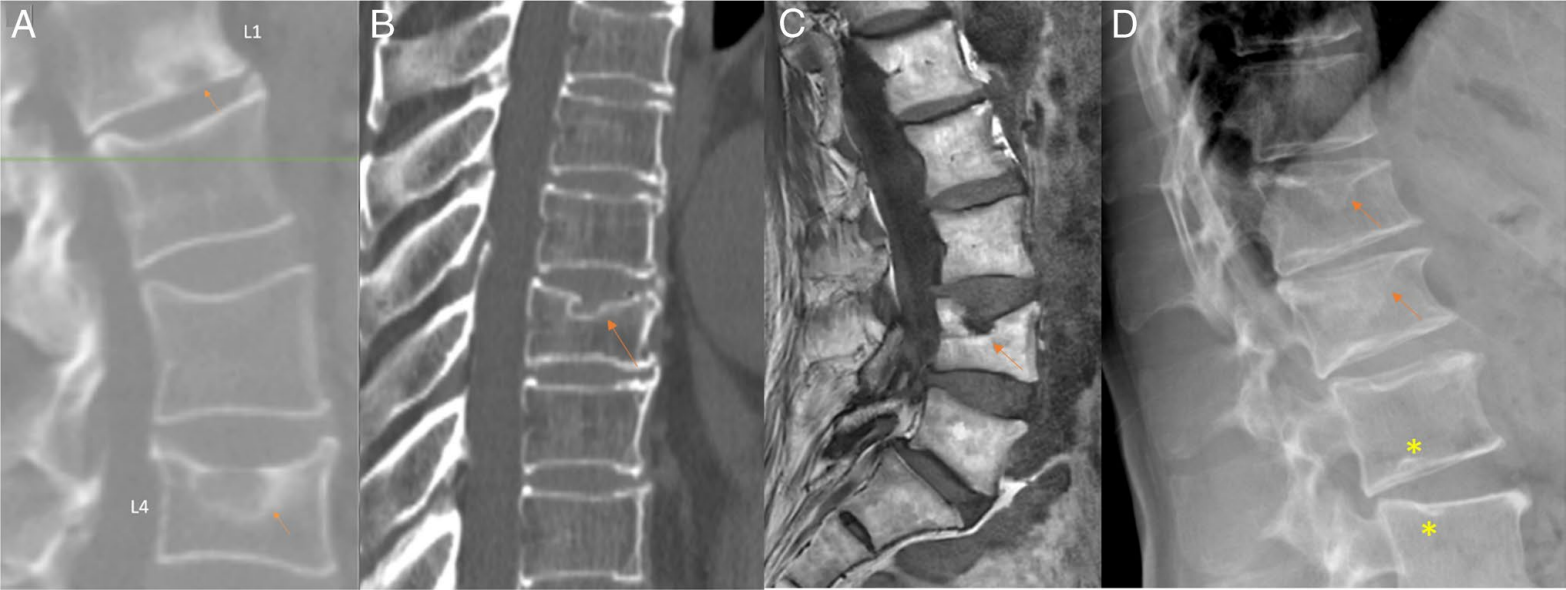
Image examples of Schmorl’s nodes of acquired cause (SNa). A Lateral radiograph. There are reactive bone changes in the inferior endplate of L1 (arrow: SNa), and a SNa in the superior endplate of L4 with an associated depression. B Reconstructed CT image. The arrow indicates the superior endplate SNa. C On T1-weighted sagittal MR image, the L4 OLVF with apparent superior endplate depression and the SNa are demonstrated. D Lateral radiograph. There is a superior endplate SNa in L1 together with OLVF, as well as a superior endplate SNa in L2 with apparent endplate fracture. The asterisks in L4 and L5 mark Schmorl’s nodes of developmental cause. OLVF, osteoporotic-like vertebral fracture. Reproduced with permission from Wáng. Quant Imaging Med Surg 2023;13:4044–4049
